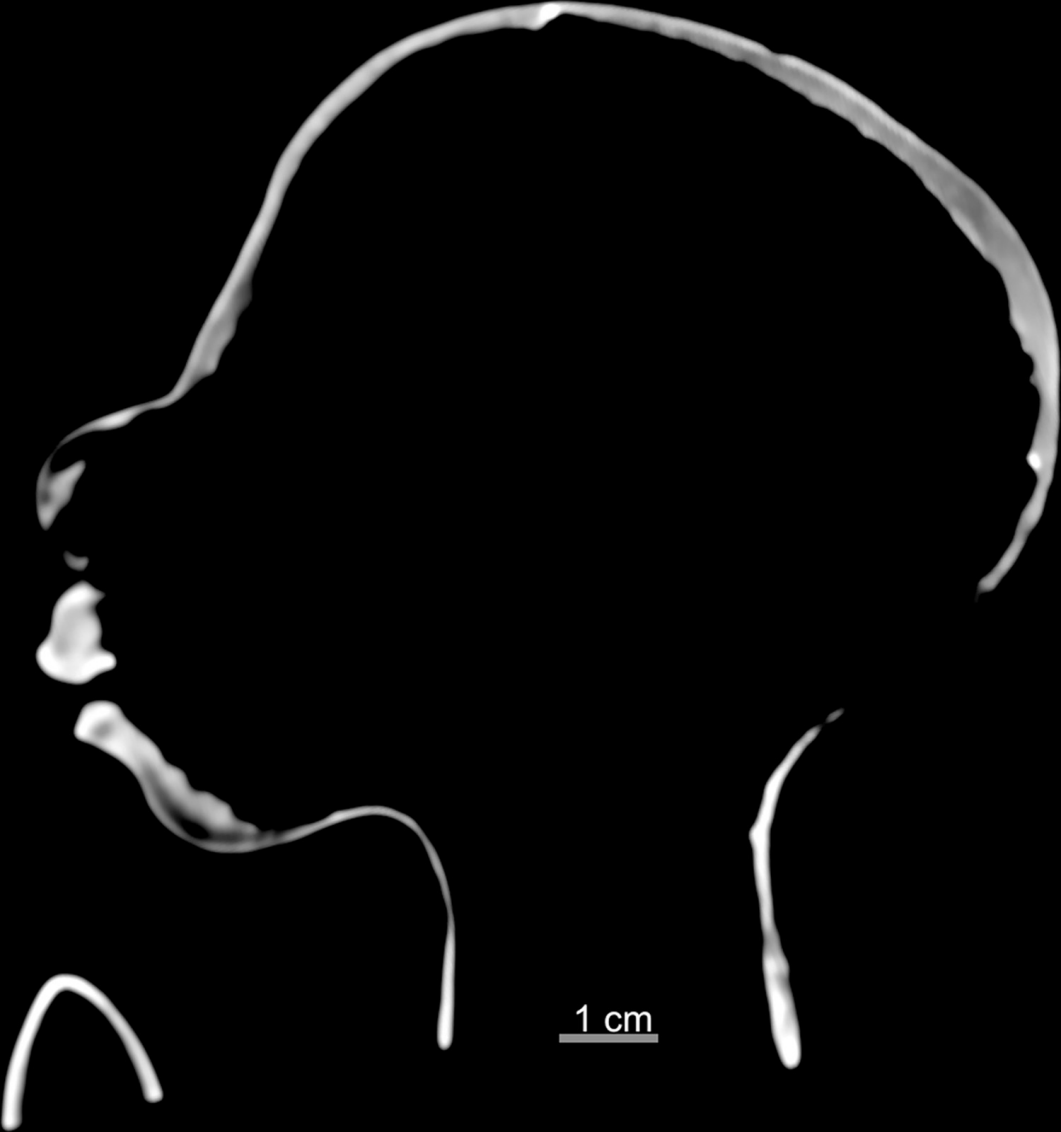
Comparative slice images of the scalp at different resolutions and magnifications. Mid-sagittal slice through the *tsantsa*–clinical CT scan. The scan has been windowed and leveled to maximize the visibility of the double hiding on the posterior part of the scalp.
